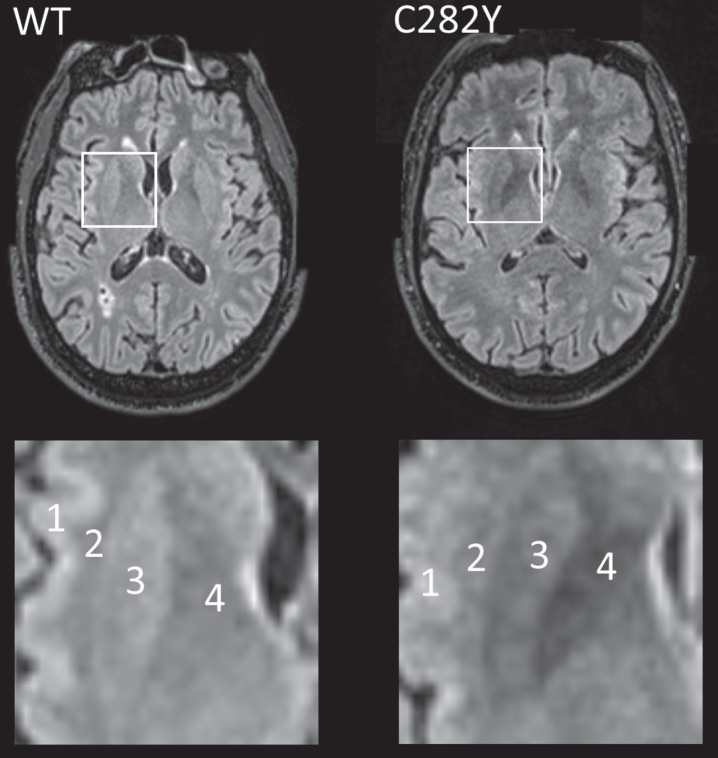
MRI T2 FLAIR axial images for male participants closest to respective mean putamen T2^*^ values with p.C282Y homozygote variants (right) and neither *HFE* variant (left, ‘WT’). In the highlighted square, cortical (1) and white matter (2) intensity appears similar in both images, but the p.C282Y homozygote image shows relative hypo-intensity (associated with iron deposition) in the putamen (3) and globus pallidus (4). Images provided by UK Biobank copyright under license. WT, wild type or neither p.C282Y nor p.H63D mutations present. See details of image acquisition in Supplementary Material.
